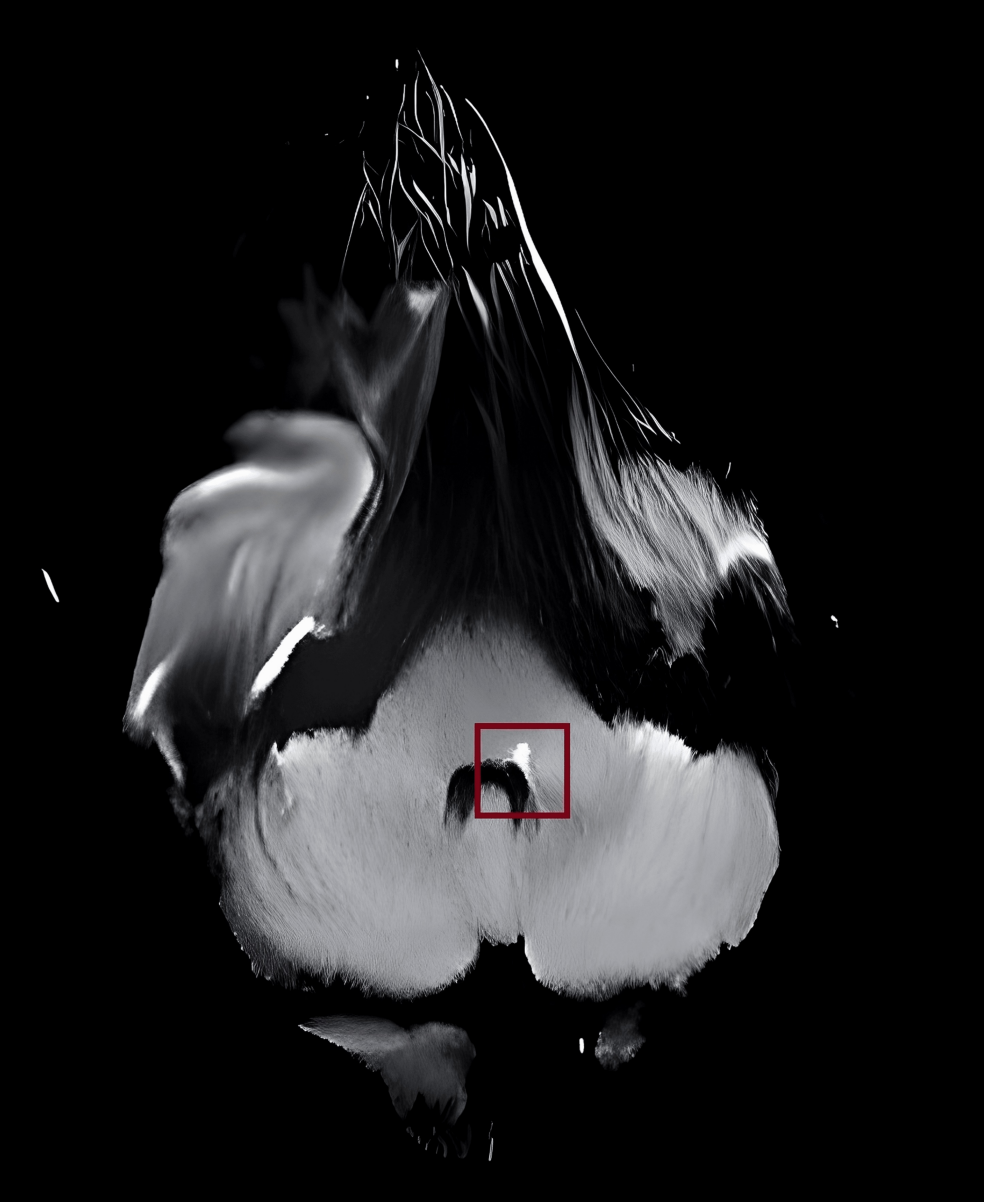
Diffusion-weighted MRI showing hyperintense foci at the junction of left lateral pontine tegmentum and left middle cerebellar peduncle MRI: magnetic resonance imaging
